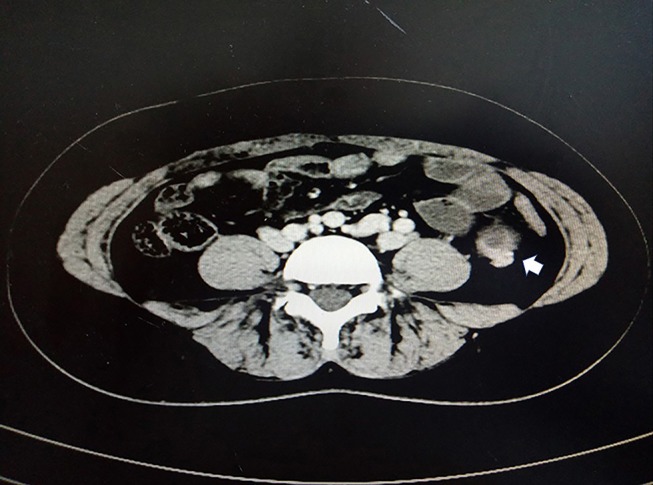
Contrast-enhanced computed tomography (CT) showing a cystic mass in the left lower quadrant (white arrow).
